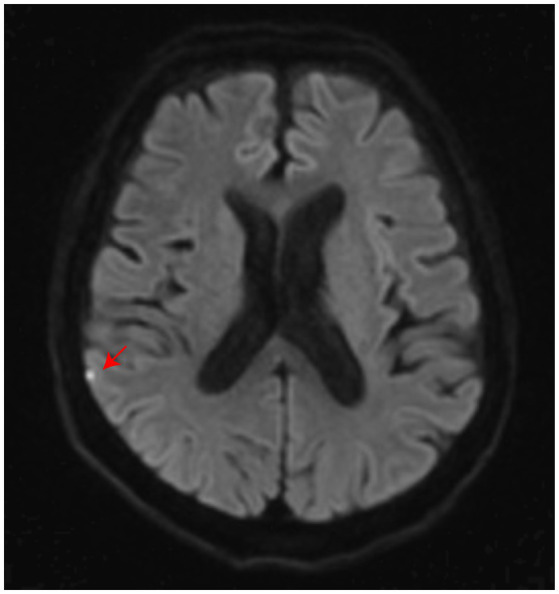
Brain magnetic resonance imaging findings. Diffusion-weighted imaging showed a focal hyperintense lesion in the occipital lobe (red arrow).
